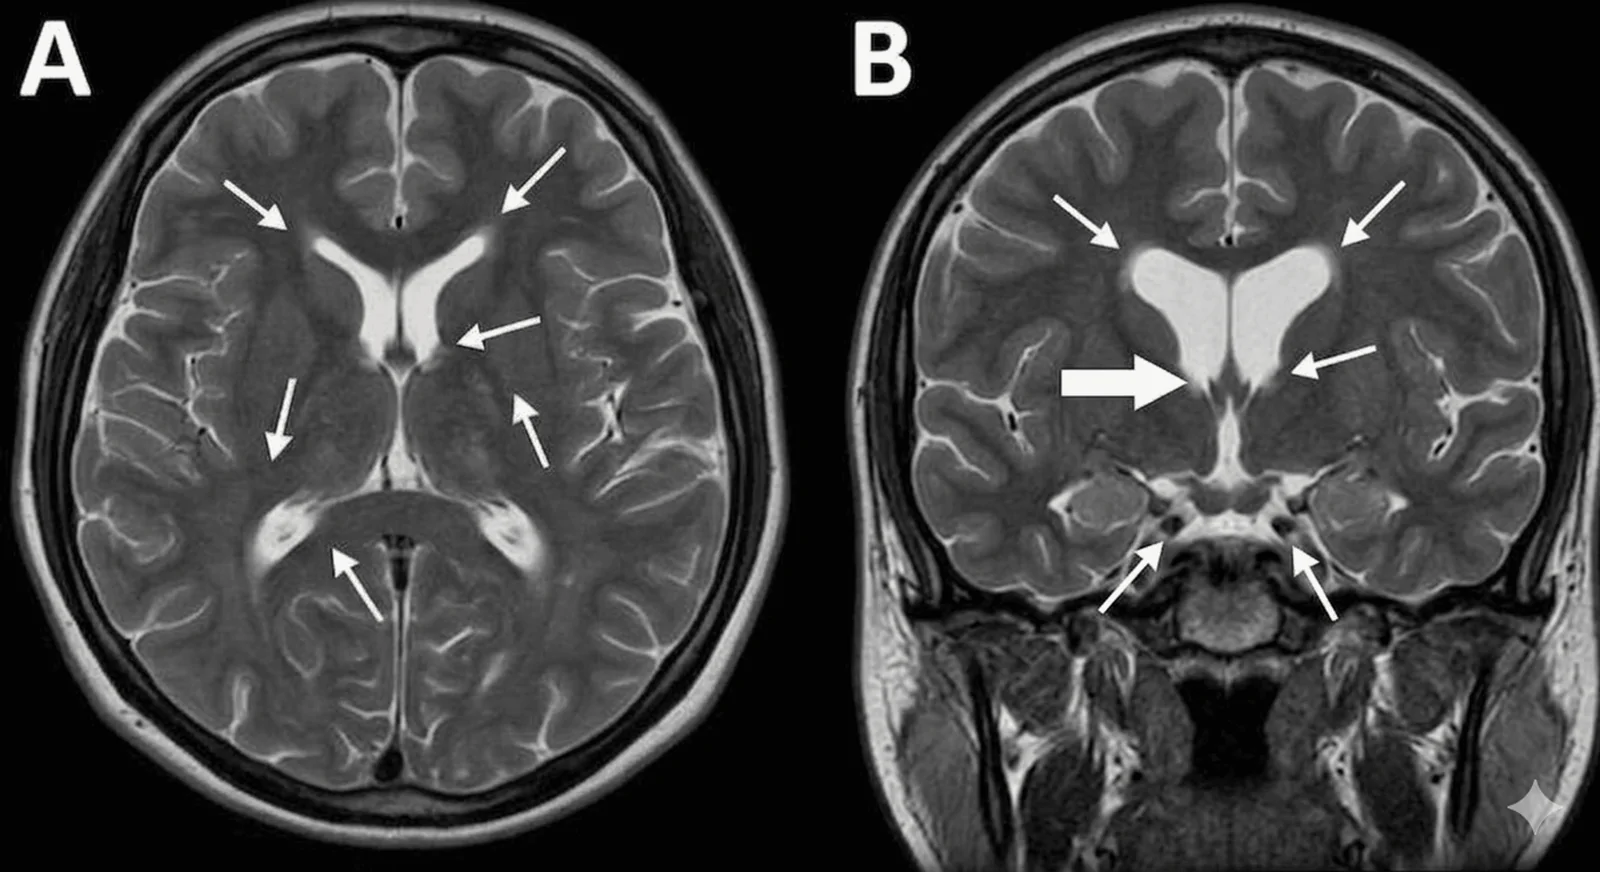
Comprehensive T2-weighted axial MRI analysis. Brain MRI findings confirming septo-optic dysplasia. (A) The axial T2-weighted sequence image shows preserved cortical and deep gray matter structures, highlighting the bilateral prominent ventricles (arrows). (B) A complementary slice demonstrates the characteristic absence of the septum pellucidum and structural midline anomalies, establishing the diagnosis of De Morsier syndrome. The thick white arrow indicates the absence of the septum pellucidum, resulting in characteristic communication and fusion of the lateral ventricles across the midline. The thin white arrows indicate bilateral hypoplasia of the optic nerves (lower arrows) and secondary dysmorphic dilation of the frontal horns of the lateral ventricles (upper arrows).
